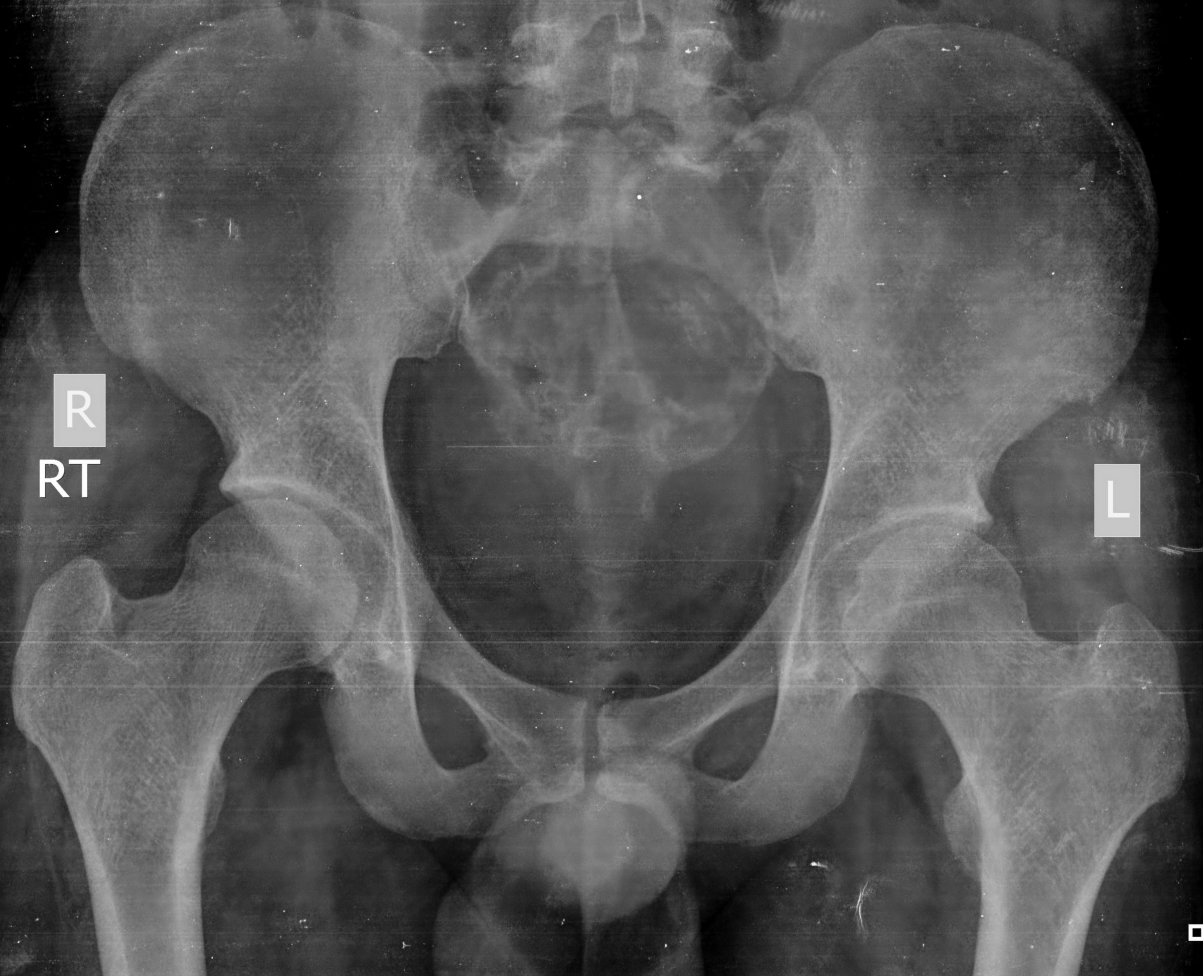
Anteroposterior radiograph of the pelvis showing patchy calcification and ossification with peripheral sclerosis of the tumor, indicating improvement after denosumab therapy (Case #2).
